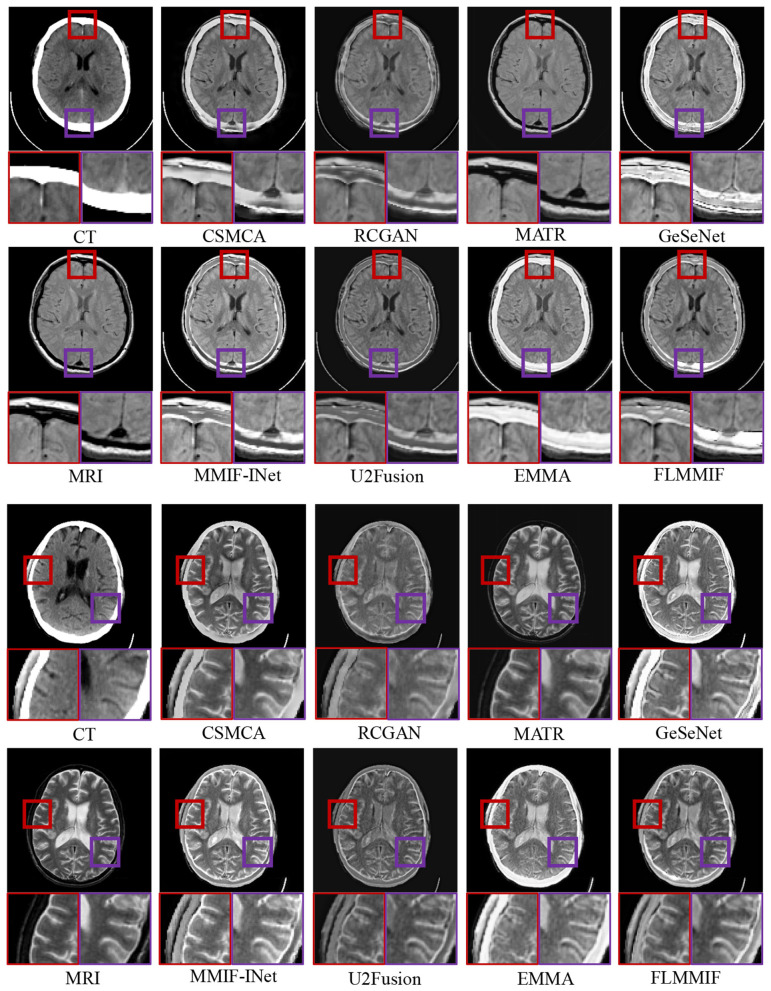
Qualitative assessment on CT-MRI dataset.
